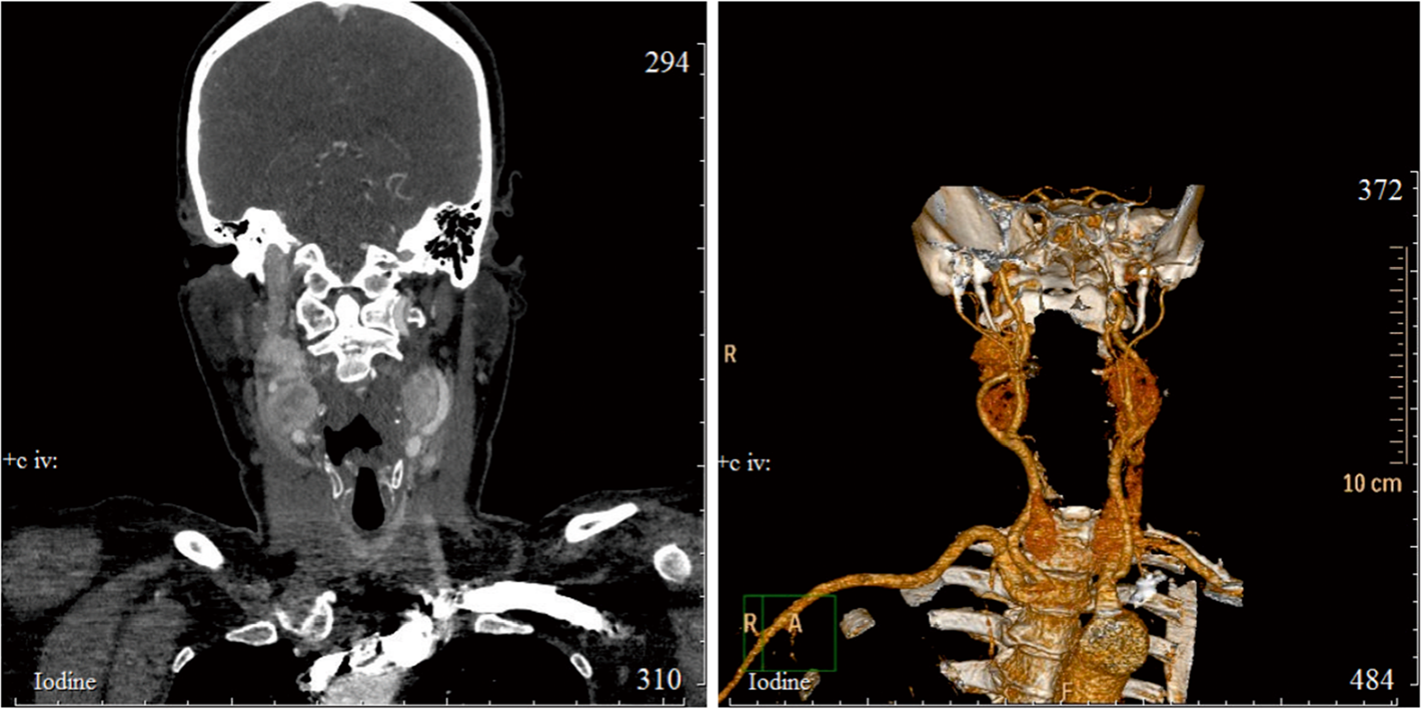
Computed tomography of the patient's head and neck before surgery. CT scan (left); 3D reconstruction (right)
